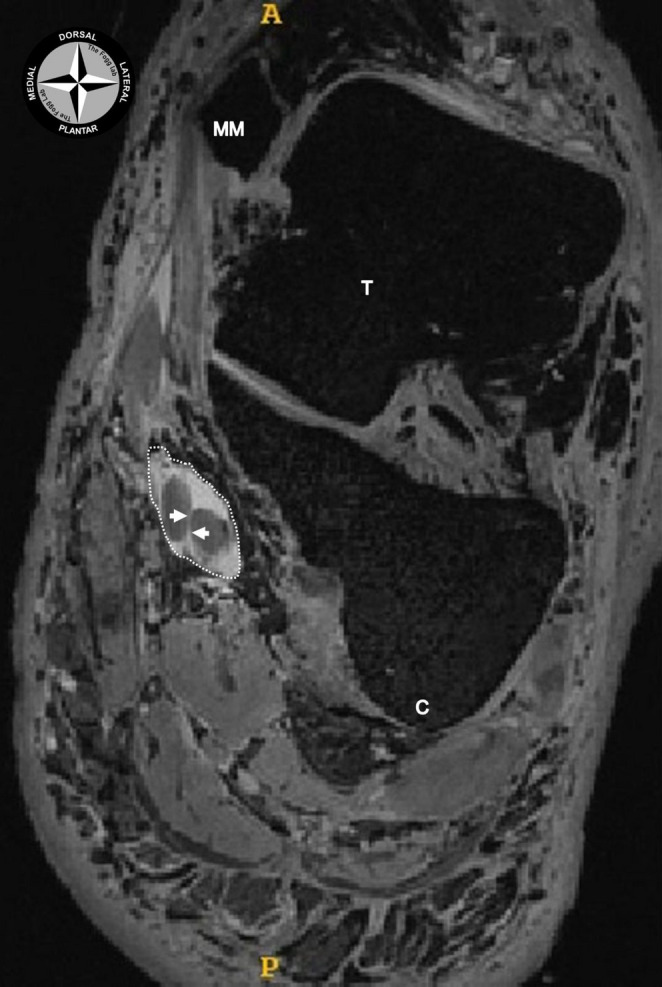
A coronal section of an MR image at the level of the chiasma plantare, with the tendons of the flexor digitorum and hallucis longus tendons crossing over. The tendinous sheaths of these tendons were injected with Magnevist to enhance the visualisation of their spatial relationship. At this level only a thin membranous tissue (white arrows) was separating the two tendons as they coursed in their common tendinous sheath (white dotted circle). MM: medial malleolus; C: calcaneus; T: Talus
